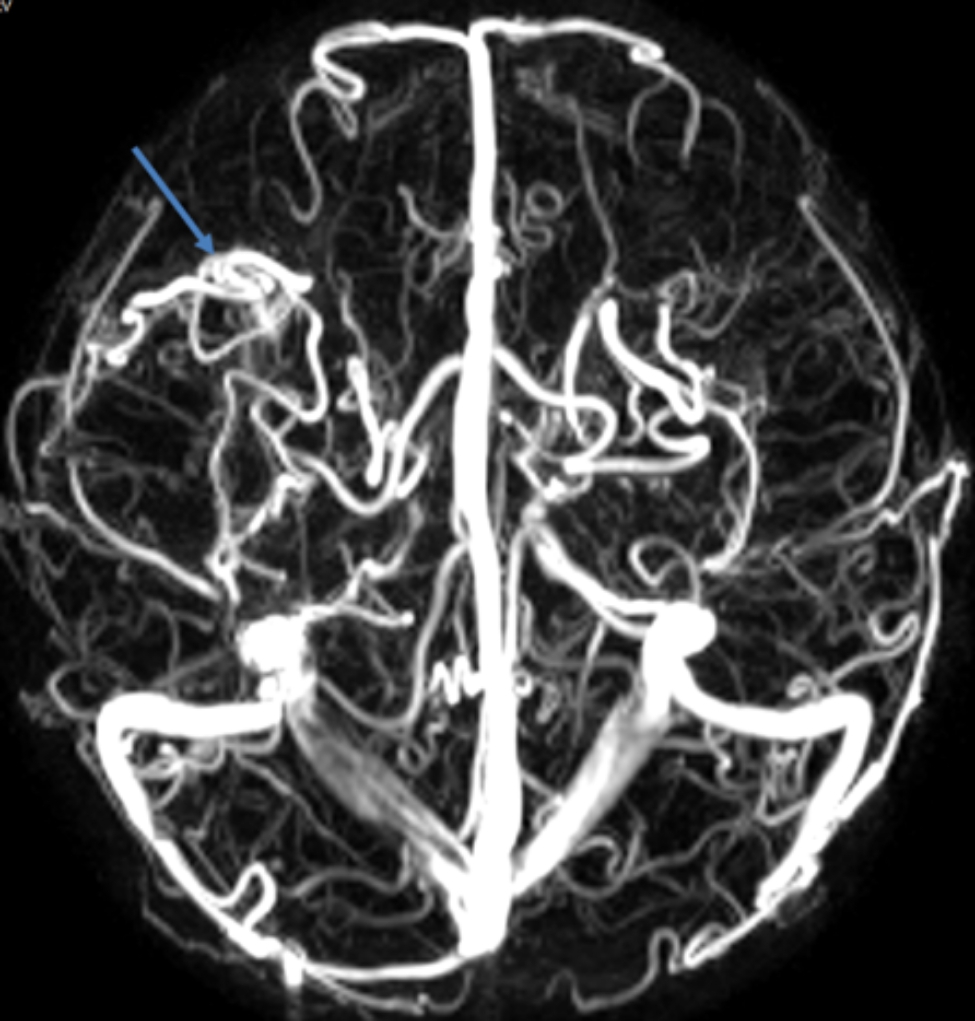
Non-contrast magnetic resonance imaging, artery and vein of the brain revealed multiple malformations of venous sinuses
